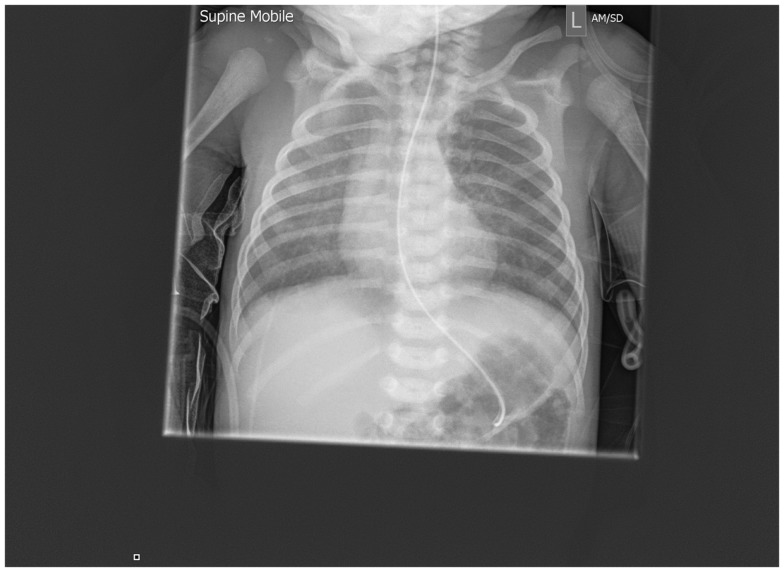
Repeat chest radiograph 1 day after admission demonstrating resolution of the changes within 24 h.
